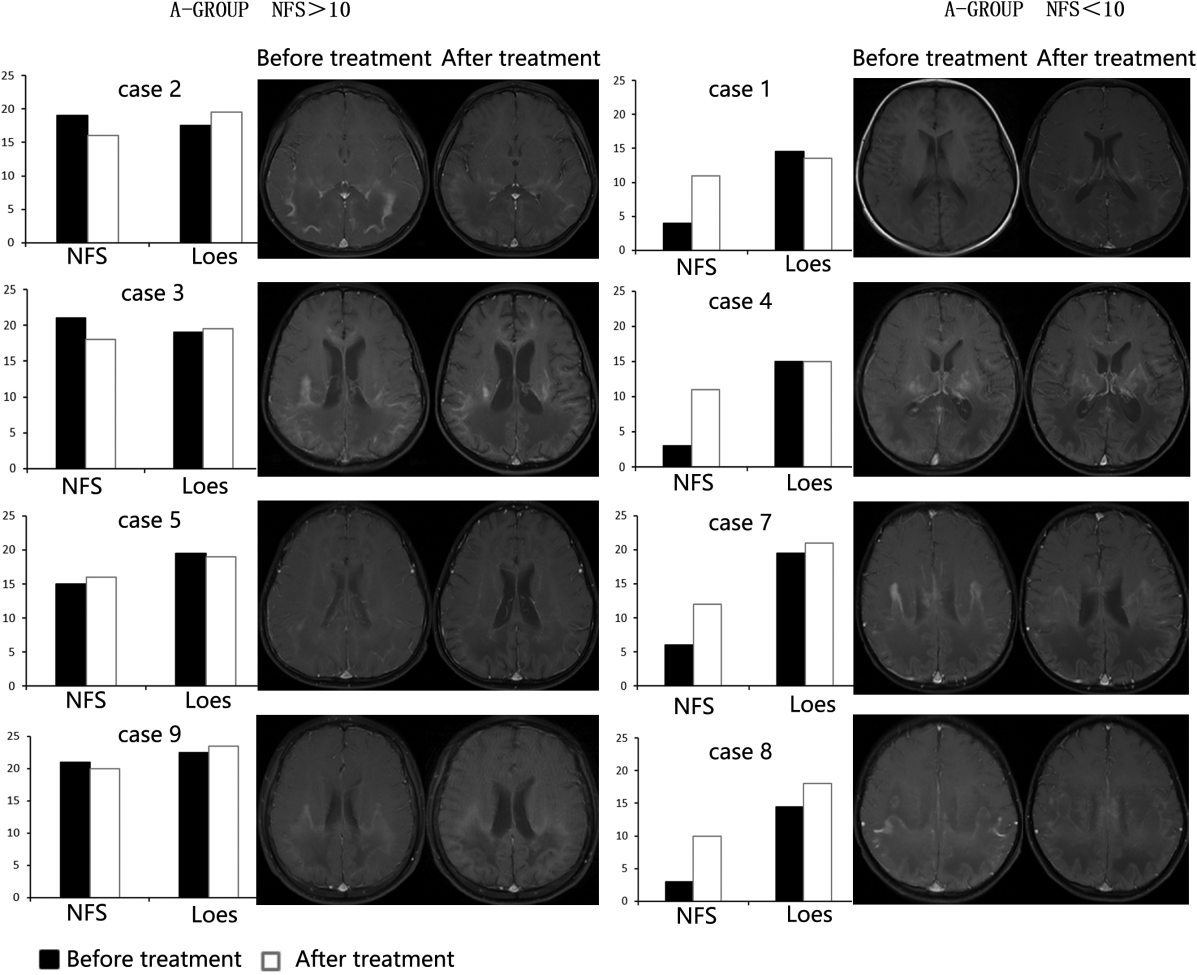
The MRI images after processed (left: before treatment, right: after treatment; case 5 could not be compared due to inconsistent scanning parameters before and after treatment and was excluded.).
